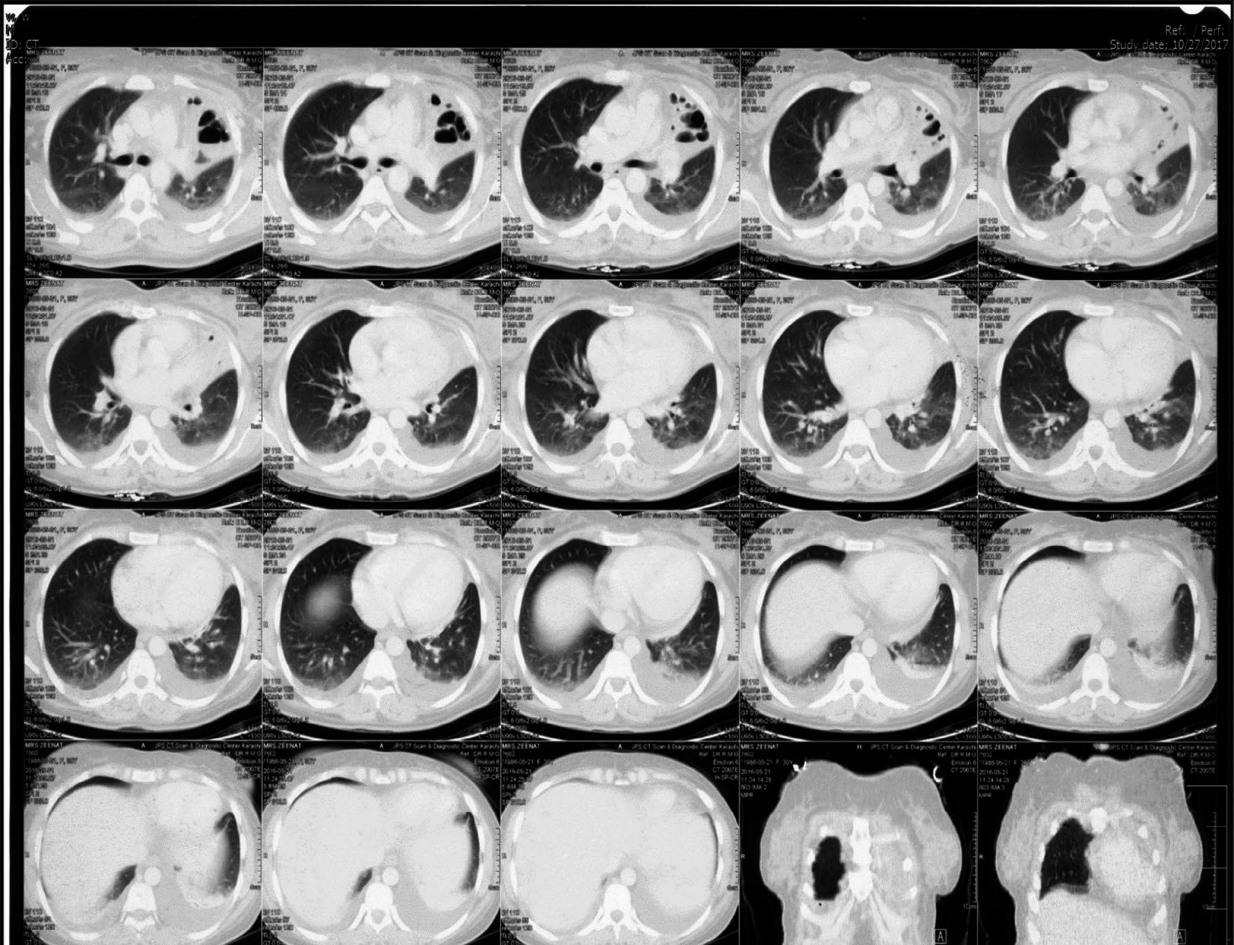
CT scan of chest with contrast redemonstrates bilateral pleural effusions and left upper lobe collapse with bronchiectasis
